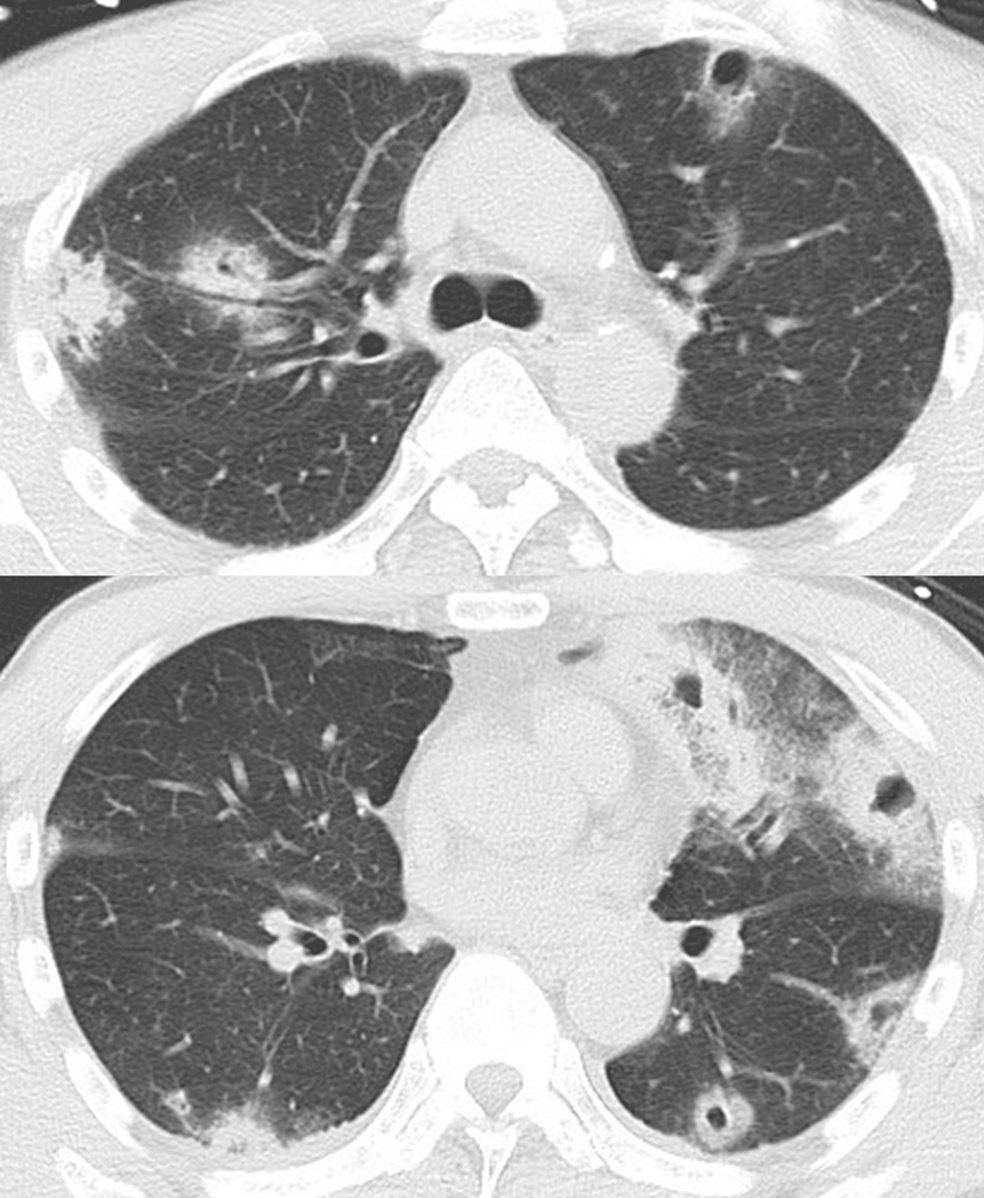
Chest computed tomography scans showed multiple bilateral nodules with cavities predominantly in the peripheral lung fields
